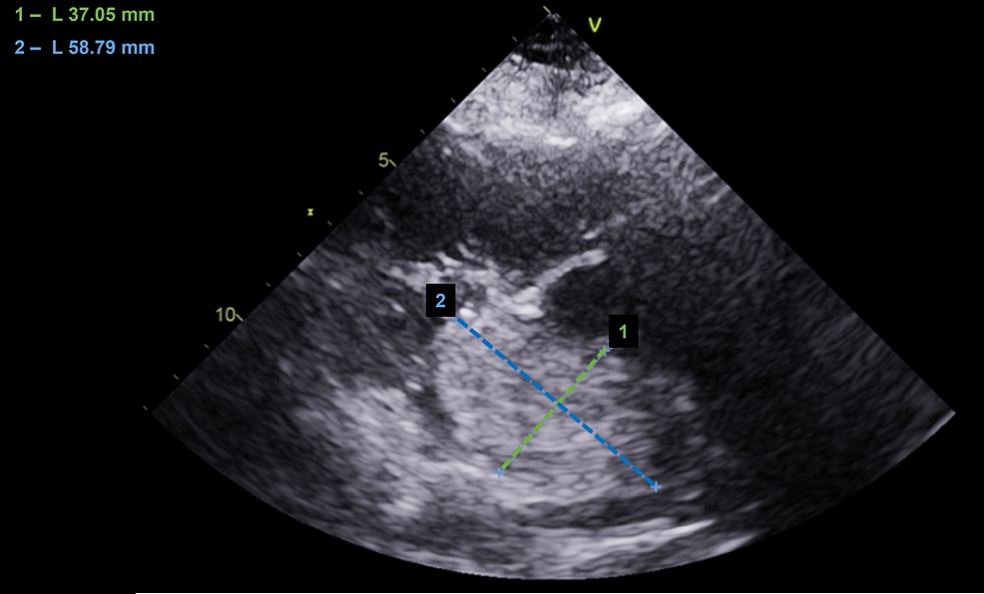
Transthoracic echocardiogram showing the left atrium filled with a mass measuring about 60x40 mm.
